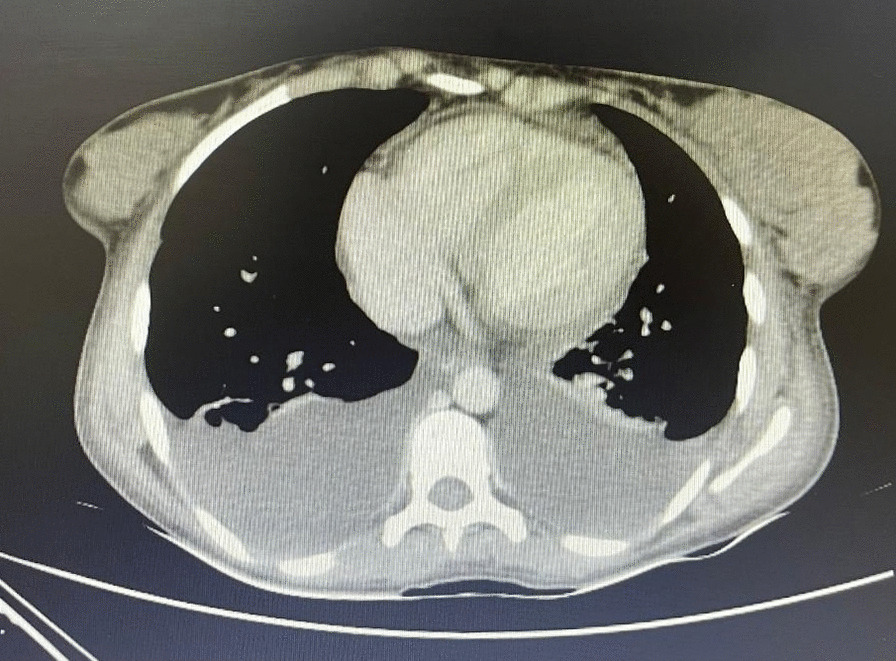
Chest computed tomography scan showing bilateral pleural effusion accompanied by mild pericardial effusion
